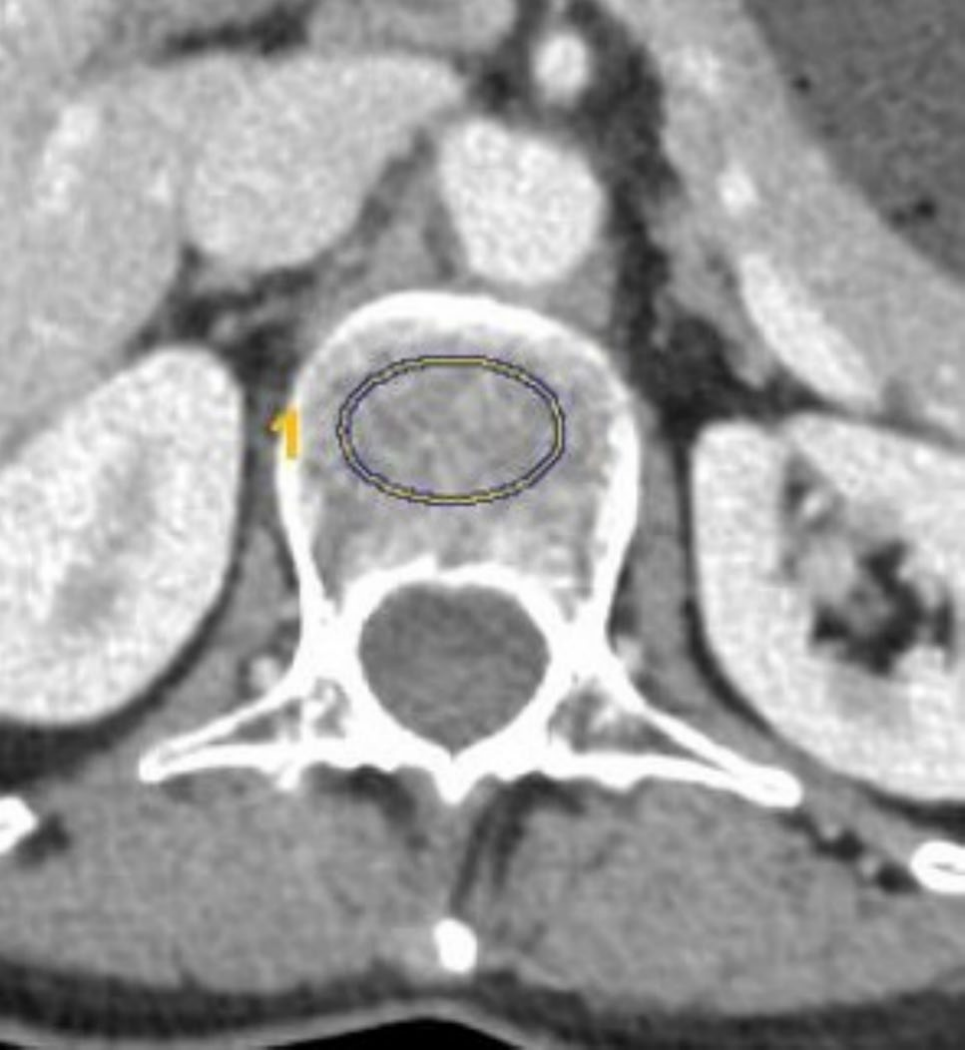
Measuring of BMD on the axial plane CT at the level of L1 vertebra
